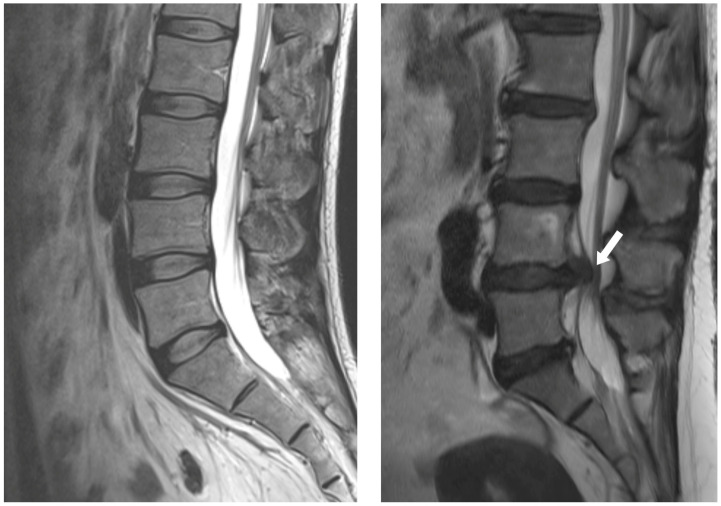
MRI images of a normal disc (left) and a herniated disc (white arrow) (right).
